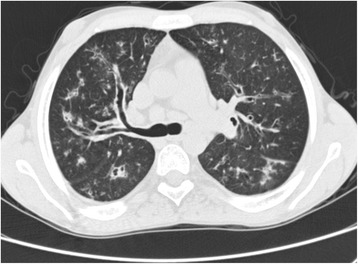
High-resolution computed tomography: cylindrical bronchiectasis, mucous plugging, and centrilobular nodules, a tree-in-bud pattern
